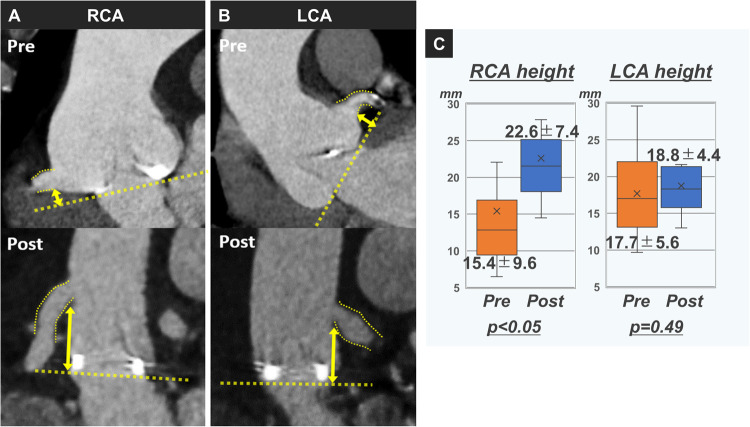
Computed tomographic data of preoperative and postoperative coronary height. (A) Reconstructed right coronary artery in a representative case of Bio-Bentall using the Piehler technique. (B) Reconstructed left coronary artery in a representative case of Bio-Bentall using the Piehler technique. (C) Comparison of the coronary heights before and after Bio-Bentall. LCA, left coronary artery; RCA, right coronary artery.
